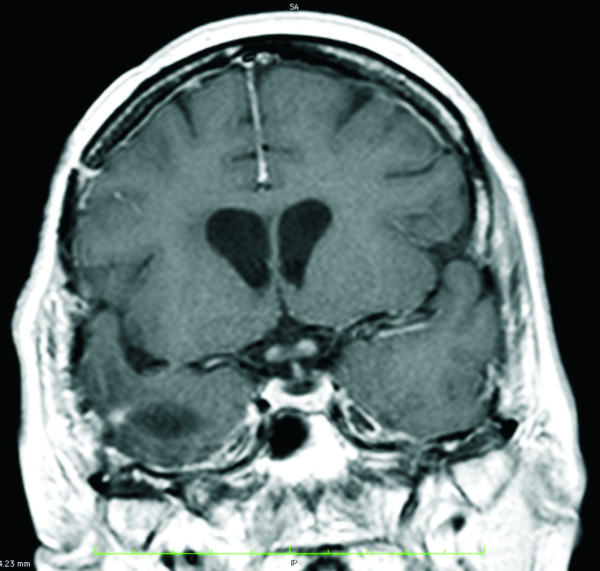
Images 5 months after cessation of radiation. T1 weighted gadolinium enhanced magnetic resonance imaging revealing subtle contrast enhancement of the left and right prechiasmatic optic nerve 5 months after cessation of radiation when bilateral amaurosis occurred. Note that there is no infiltration of the chiasm by tumor recurrence.
